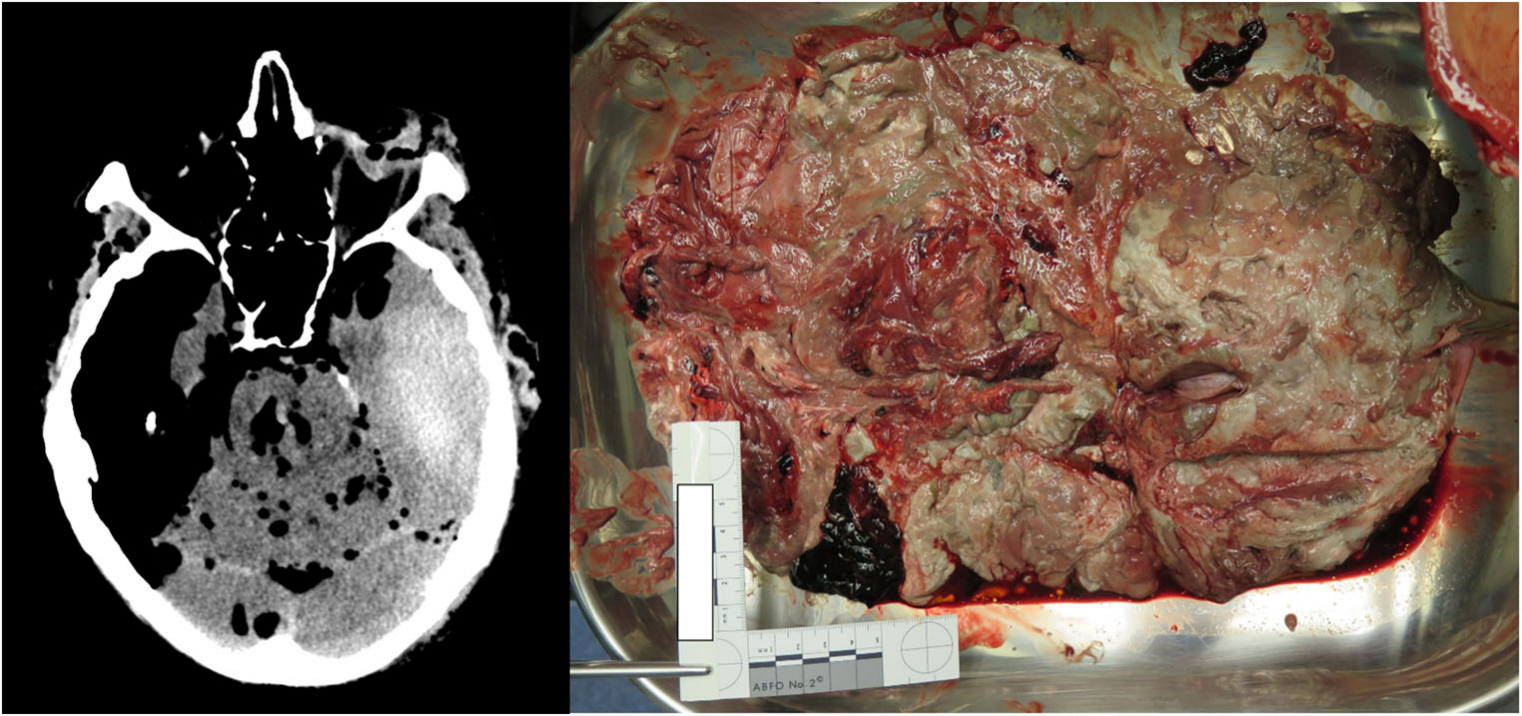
Comparison of CT results with those of autopsy, in which the brain poured out of the skull
